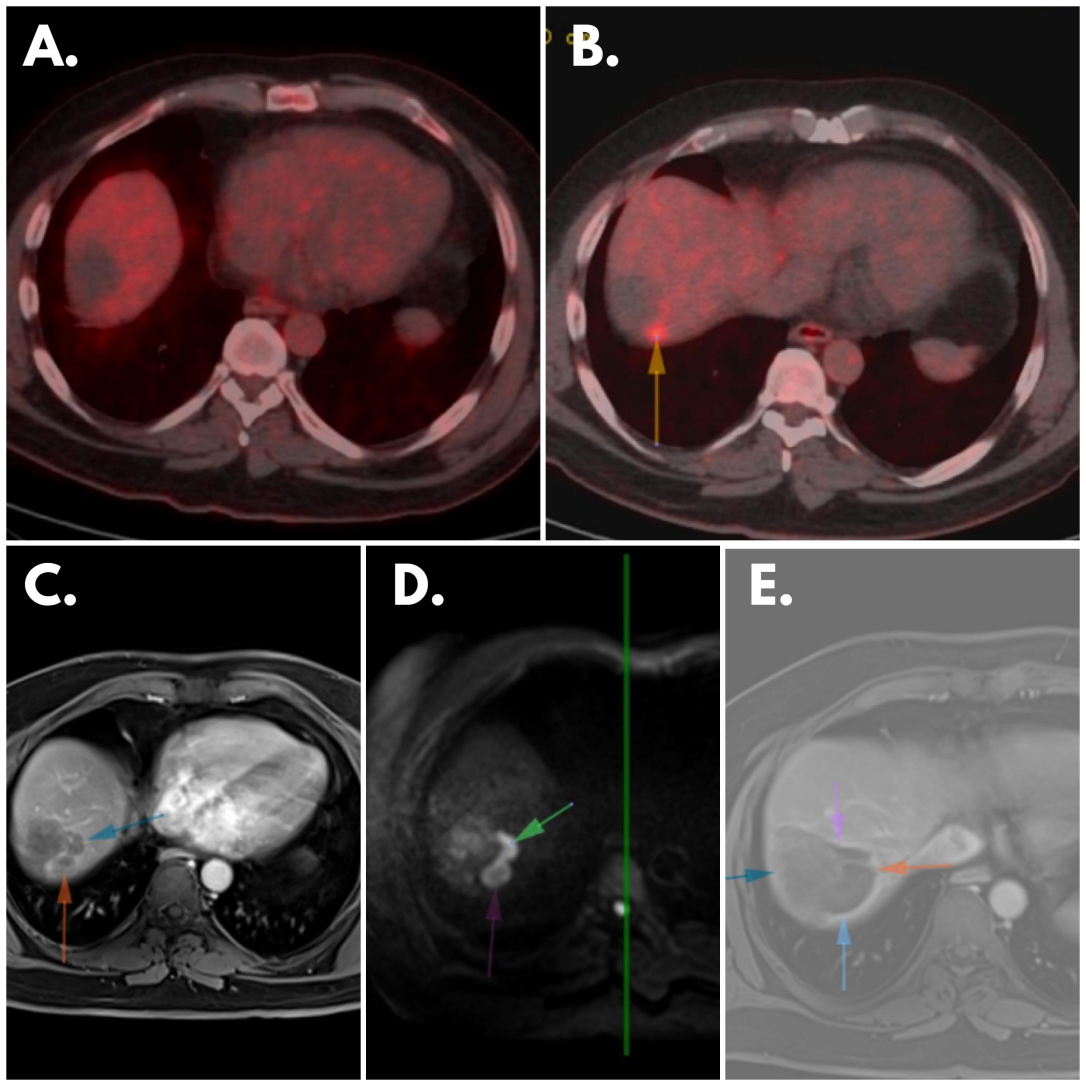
Patient’s Scannings (A) February 2023 PET: No significant FDG accumulation observed in the area of the hypodense lesion indicating lack of metabolic activity. (B) May 2023 PET: Newly developed focal FDG uptake focus (arrow) evident in immediate posterosuperior neighborhood of previously identified lesion, suggesting change in metabolic activity, indicative of disease progression. (C) May 2023 MR Scanning: Peripheral contrast-enhancing lesions visible in localization described in February 2023’s PET, suggesting presence of enhanced vascularity. (D) May 2023 MR Scanning: Diffusion-restricting lesions observed in same localization, indicating restricted water diffusion within lesions, potential marker of cellular density and tumor activity. (E) Post-treatment MRI: Cavitary area without contrast enhancement and diffusion restriction evident in lung, suggesting treatment-induced change such as necrosis or resolution of previously identified lesion, signifying favorable treatment response in this specific anatomical area.
